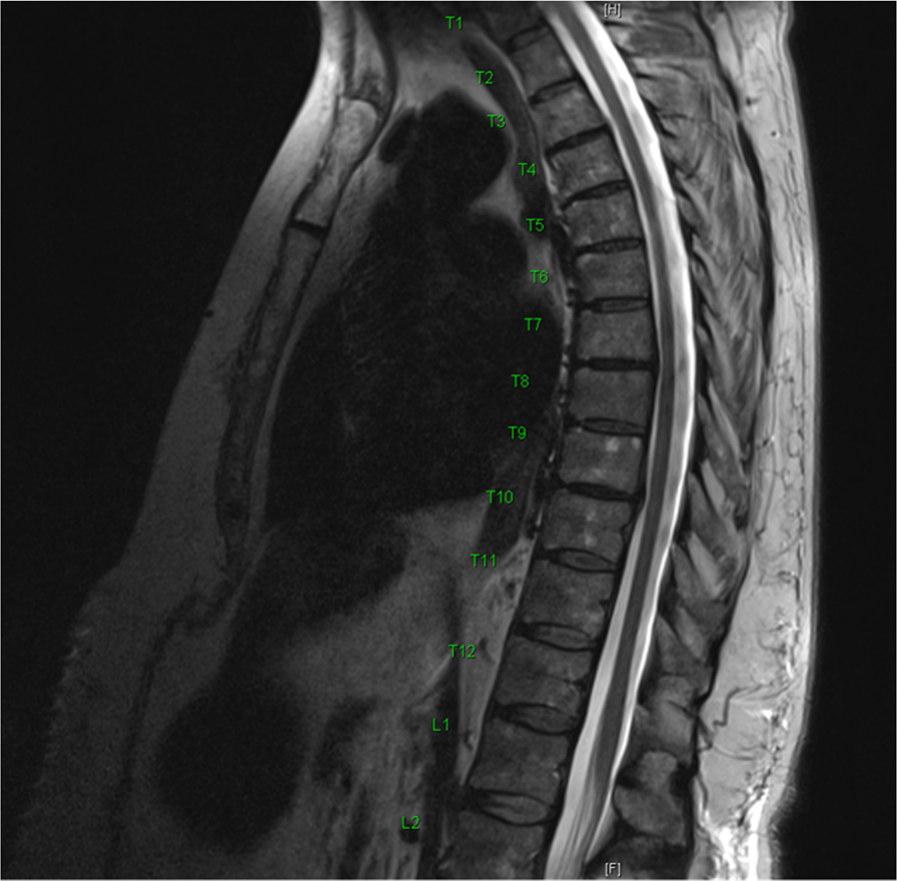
Sagittal magnetic resonance imaging of the thoracolumbar spine on day 5 after the abdominal surgery and epidural anesthesia. Note the absence of canal compromise, edema or bleeding.
